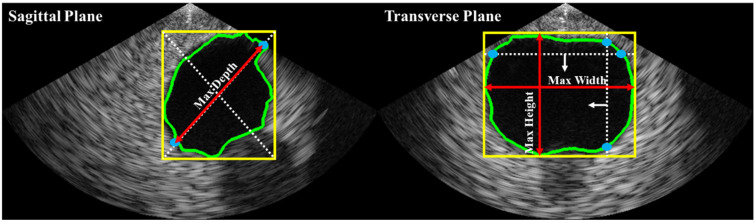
Automated axes detection for estimating bladder height, width, and depth from biplane ultrasound images. The bladder ROI dimensions were obtained by calculating the minimum enclosing bounding box (yellow line). In the sagittal plane, the greater distance between two intersections (blue dots) of the bounding box’s diagonal line (white dashed lines) with the bladder ROI contour (green line) was used to estimate depth. The red arrow lines represent the estimated depth. In the transverse plane, the maximum distances of the vertical and horizontal intersections were used to estimate height and width, respectively.
